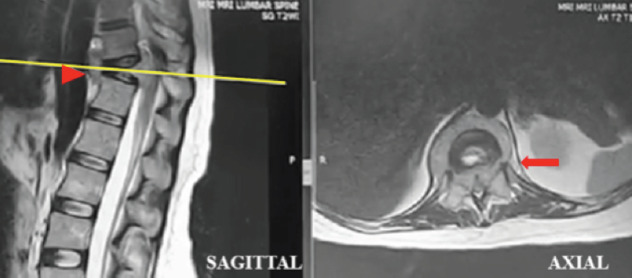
Magnetic resonance imaging (MRI) of the patient’s spine. Arrowhead shows T11 vertebra plana with kyphotic deformity. Arrow demonstrates paravertebral soft tissue mass formation extending into spinal canal causing spinal canal stenosis and cord compression.
